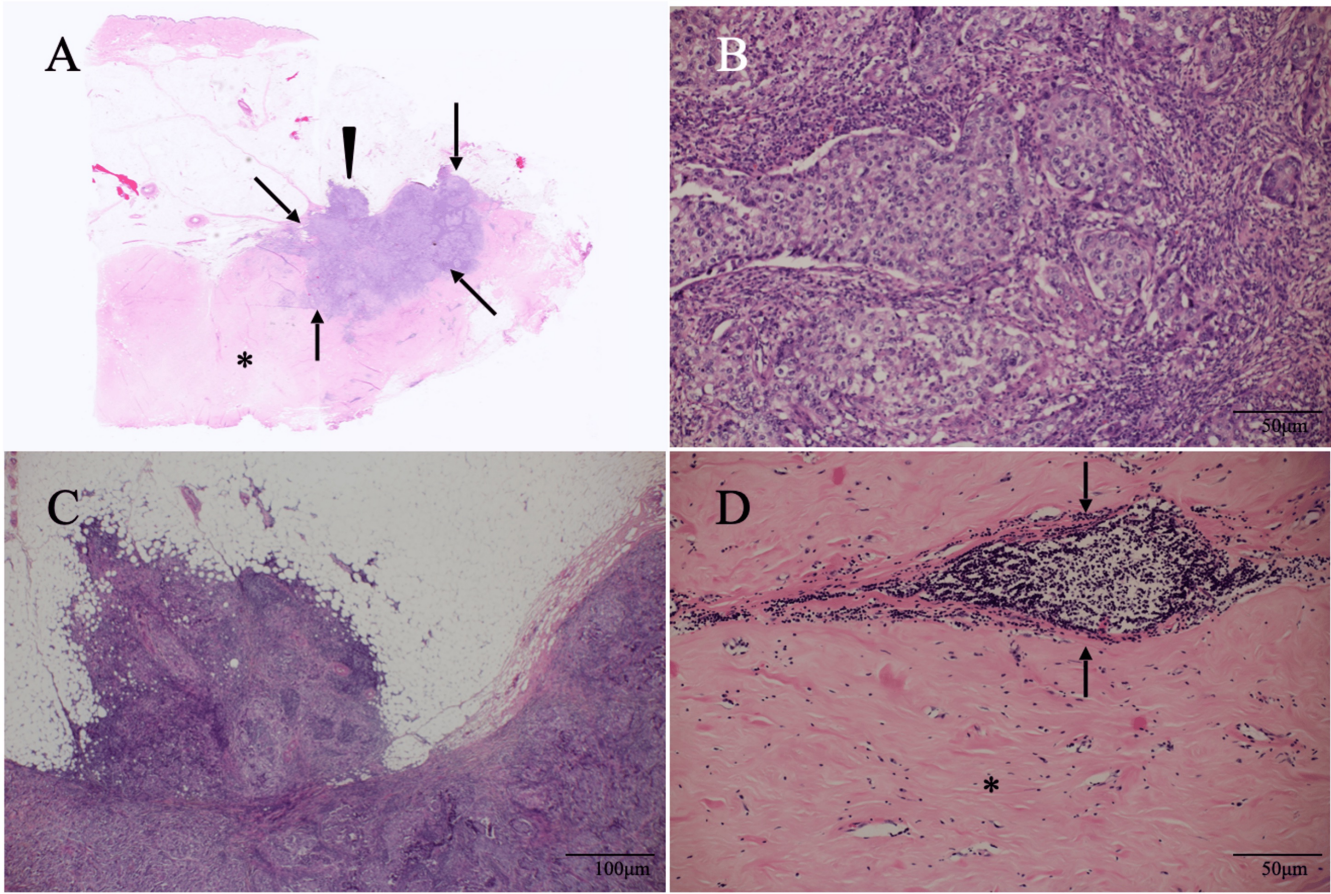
Pathological findings A. Low magnified view showed an irregular mass (arrows) with fat invasion (arrowhead) in the fibrous disease (asterisk). B. Magnified view showed atypical cells growing in an expansive fashion. C. Magnified view showed the fat invasion areas, well corresponding to the disruption of the anterior margins of the mammary gland on ultrasound. D. Magnified view showed abundant collagen fibers (asterisk) and peri-ductal inflammatory cell infiltration (arrows).
